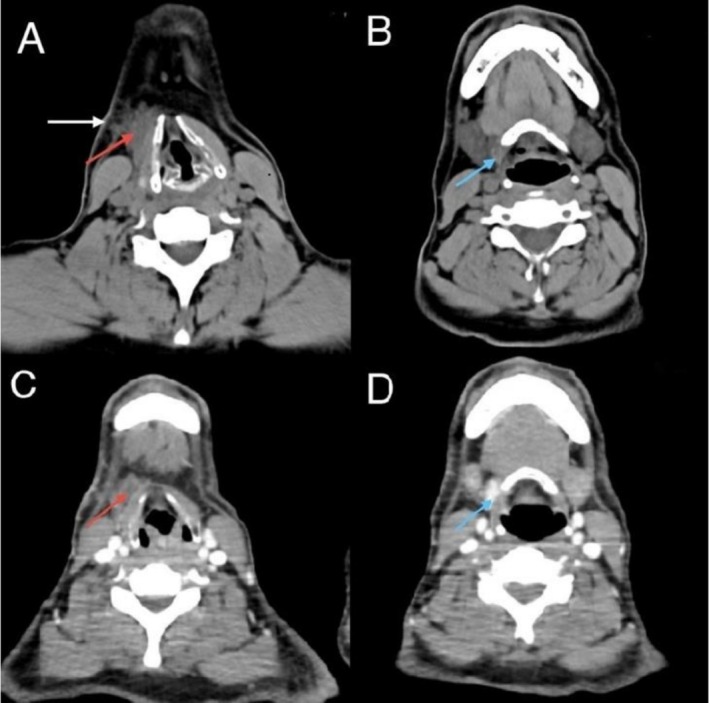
Axial neck CT images without iodinated contrast (A, B) and with iodinated contrast (C, D) showing a paramedline infrahyoid cystic lesion (red arrow) with a hyperdense solid enhancing component (blue arrow) surrounded by fat stranding (white arrow). A neck CT scan revealed a paramedline infrahyoid lesion embedded in the right infrahyoid muscle. It had cystic and solid components, no calcifications, and was surrounded by fat stranding. The presumed diagnosis was an infected Thyroglossal duct cyst with ectopic thyroid tissues.
